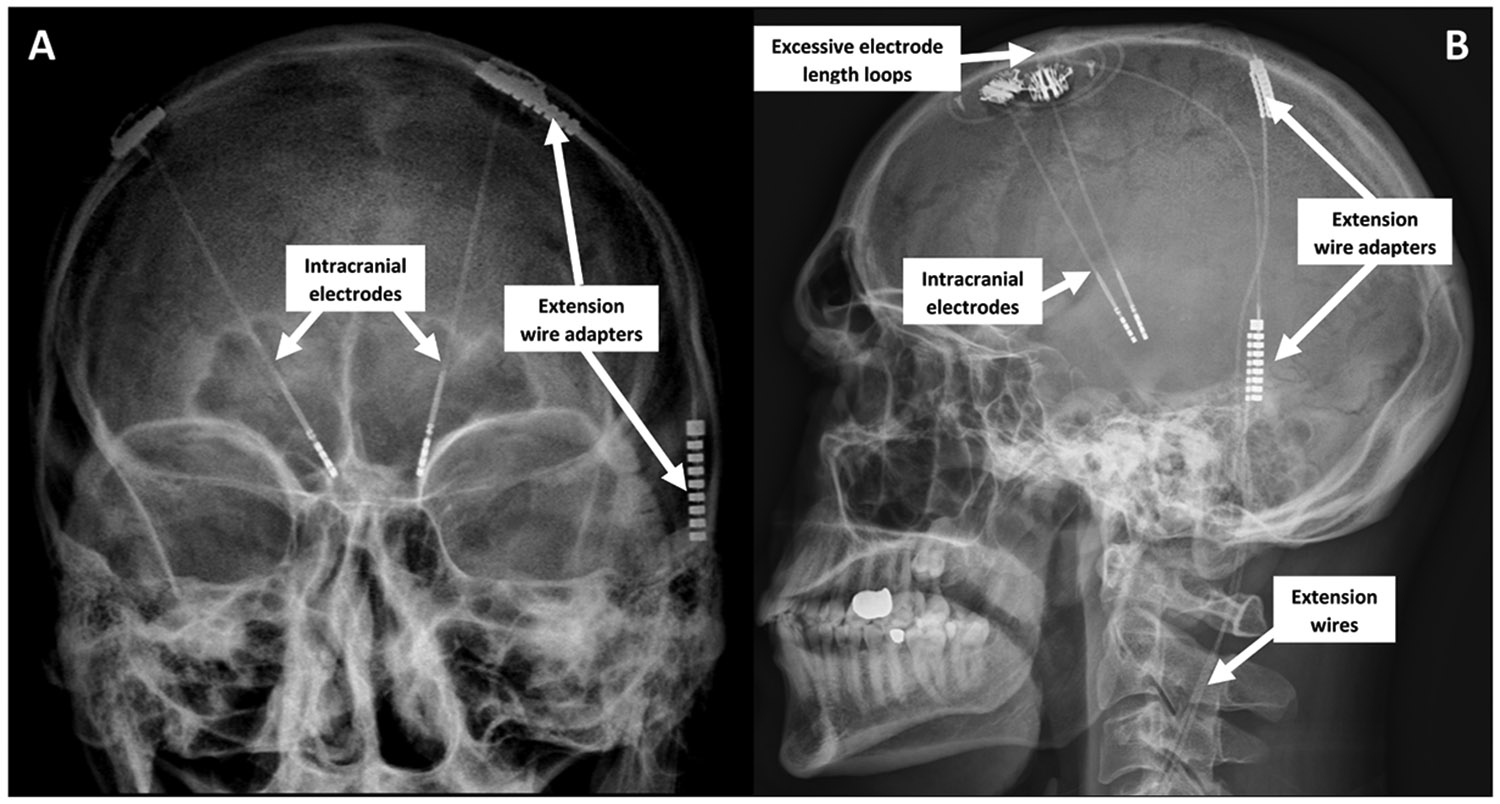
X-ray scan of a patient with implanted DBS system showing the full intracranial (leads) and scalp region hardware configuration (loops of excessive electrode length, adapters and extension wire), as possible sources of susceptibility artefacts. A – anteroposterior and B – lateral view.
